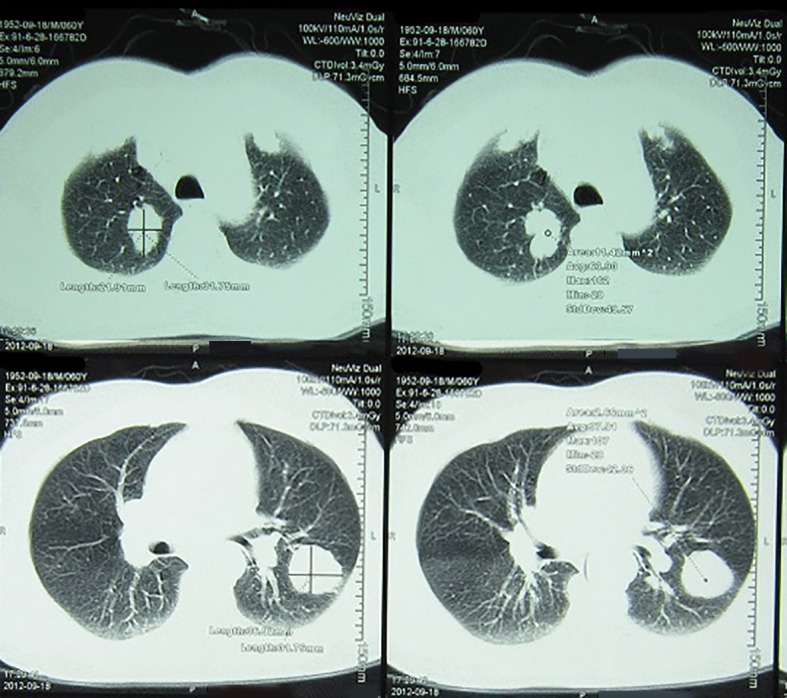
Shows the chest CT scan of the patient before starting chemotherapy with docetaxel.
